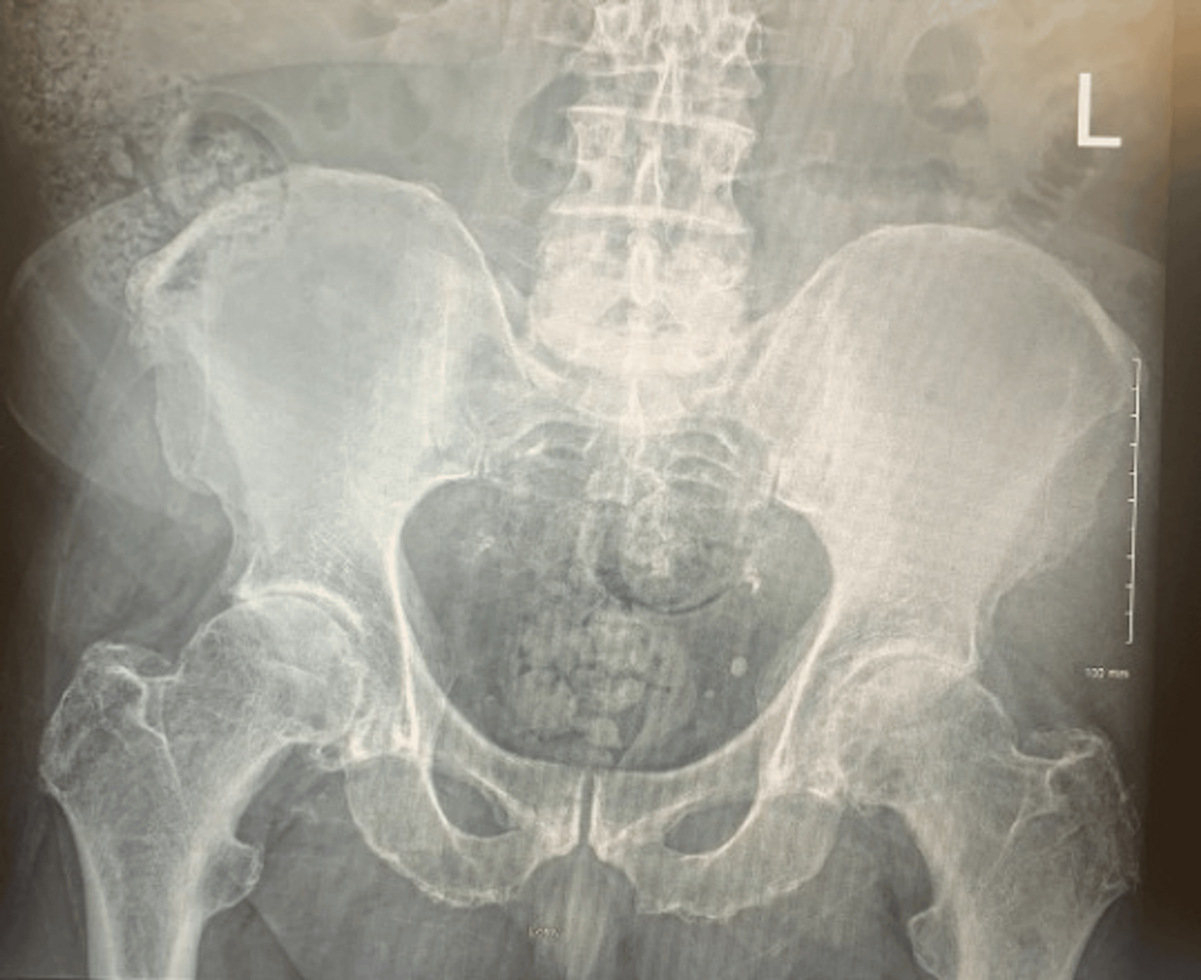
Preoperative anteroposterior radiograph of the pelvis
